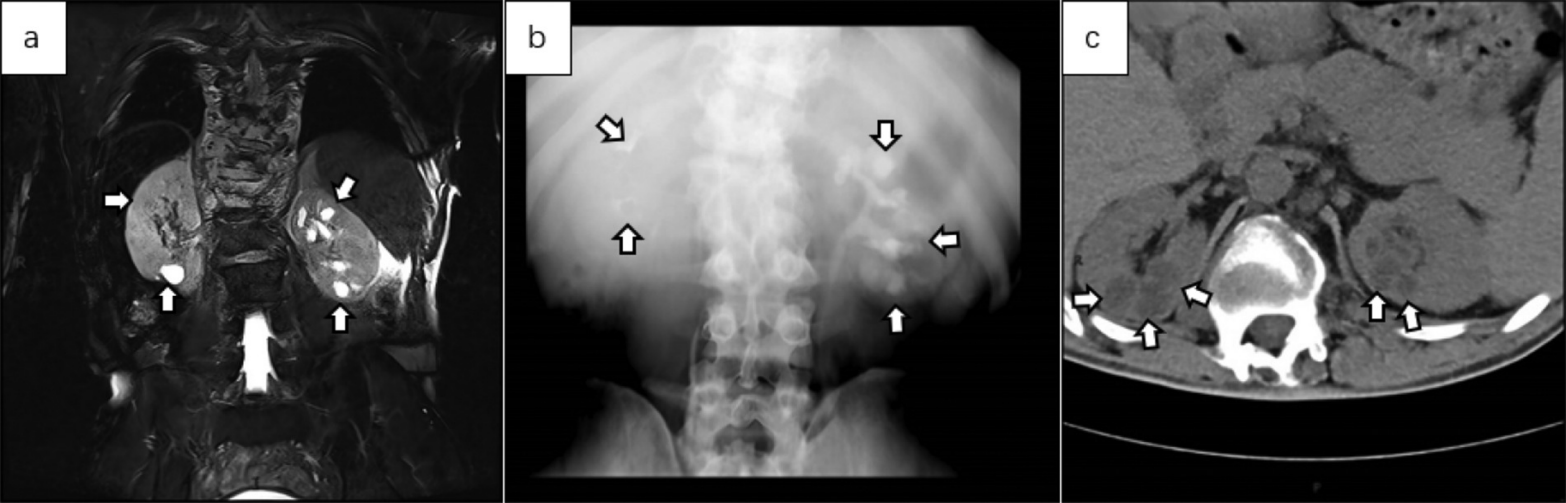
(a) coronal abdominal MRI T2WI showed multiple cystic high-signal areas in both kidneys, predominantly in the left kidney. The capsule exhibited a uniform signal, and no obvious morphological abnormalities were noted in either kidney. (b) IVU revealed contrast filling of the renal capsule in the left kidney, with expansion of the left calyx, poor contrast visualization of the right calyx and renal pelvis, and normal development of both ureters. (c) Plain axial CT images of the abdomen showed no obvious abnormalities in the morphology of either kidney, slight dilation of the upper segments of both ureters, and multiple cystic low-density shadows in the renal parenchyma.
